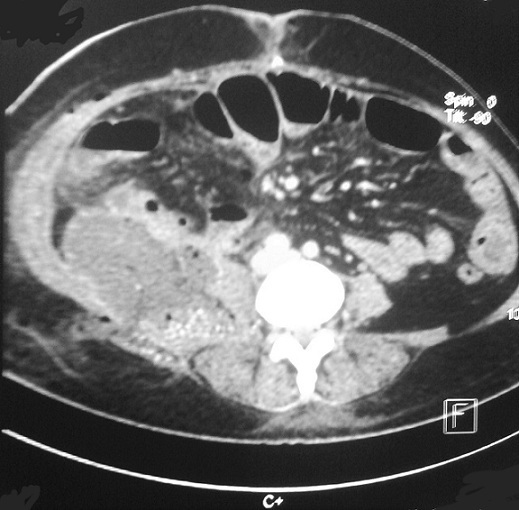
Computed tomography (CT) scan revealed a fluid collection in the psoas, associated with inflammatory changes in the local fat and a small amount of free liquid in the right parietocolic area
